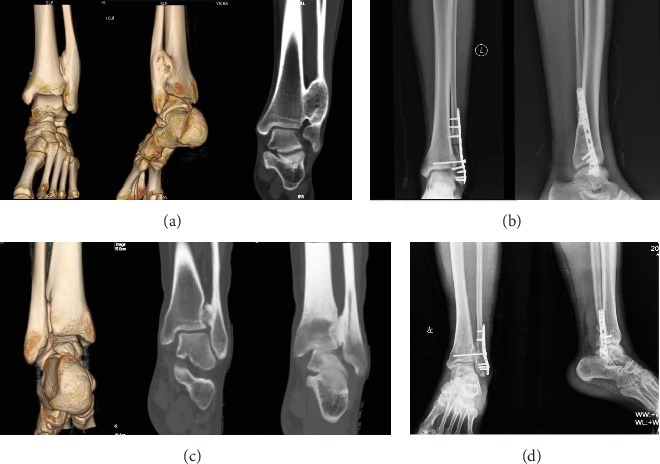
Preoperative ((a) CT scan with three-dimensional reconstruction and one layer) and postoperative ((b) anteroposterior and lateral X-ray) radiographs from a 29-year-old woman. Preoperative ((c) CT scan) and postoperative ((d) anteroposterior and lateral X-ray) radiographs from a 24-year-old man.
